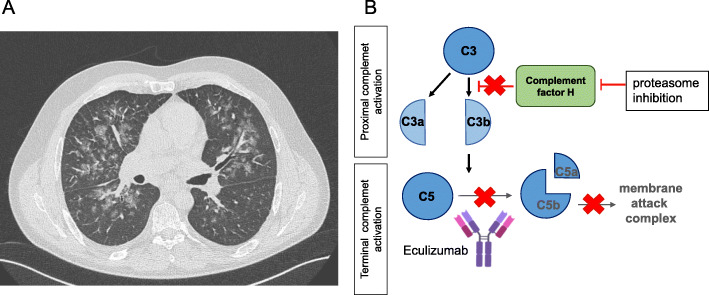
a Chest-CT in patient case 1, d1 of CFZ-TMA onset: Bipulmonary extensive ground-glass opacities in all lung fields, mostly omitting the periphery and predominantly occurring in the right lung. b Mechanism of CFZ-TMA: Within the proximal complement activation C3 is cleaved by the C3 convertase to anaphylactic 3a and C3b. C3b then cleaves C5 to C5a and C5b, the latter together with C6-C9 forming the membrane attack complex (MAC) within the terminal pathway. Activity of the C3 convertase is regulated by complement factor H and regulated proteins. Proteasome inhibition blocks CFH [11]. The monoclonal antibody eculizumab counteracts the resulting overactivation of C3b by binding C5
